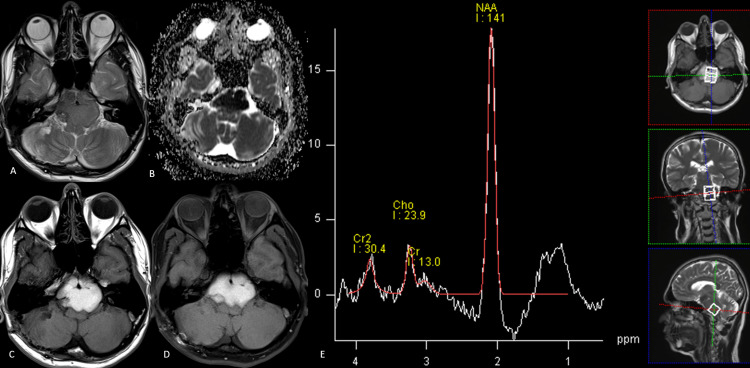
Neurenteric cyst A lobulated hypointense lesion is seen around the basilar artery, ventral to the medulla, extending into bilateral cerebellomedullary cisterns on the axial T2-weighted image (A). There is no narrowing or displacement of the vessel. ADC map (B) shows diffusion restriction. It is uniformly hyperintense on the axial T1-weighted image (C) with no loss of signal on the axial T1 fat-saturated sequence (D). The large NAA-like peak on MRS (E) supported a diagnosis of a neurenteric cyst, ruling out a white epidermoid. A lactate peak is also present. NAA: N-acetyl aspartate; ADC: apparent diffusion coefficient
